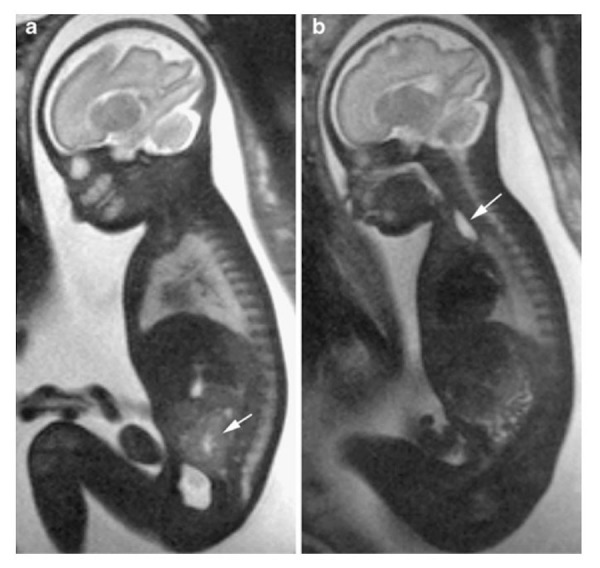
Horseshoe kidney T2-weighted HASTE imaging of a 33-week gestational age fetus. Panel A consists of a sagittal image demonstrating a horseshoe kidney (arrow). Panel B is a sagittal slice also demonstrating a horseshoe kidney and a pouch in the upper esophagus (arrow) consistent with a diagnosis of tracheoesophageal fistula. Image reprinted without change from Martin, et al. under the creative commons license.
